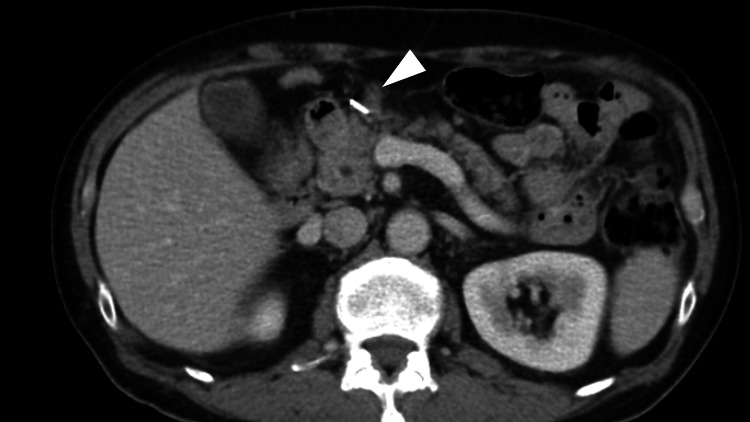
Axial abdominal contrast-enhanced computed tomography after 13 courses of pembrolizumab monotherapy The enlarged lymph nodes (arrowhead) almost disappeared after 13 courses of pembrolizumab monotherapy.
